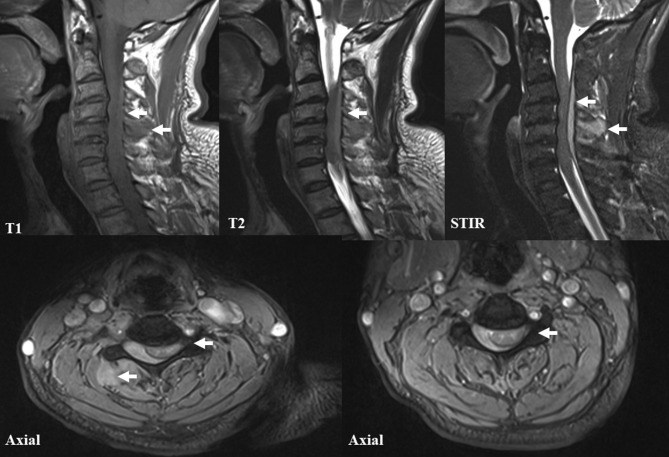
Magnetic resonance imaging (MRI) of the cervical spine. The T1-weighted, T2-weighted, short tau inversion recovery (STIR) and axial cervical MRI images demonstrated a longitudinal dorsal extradural hematoma, extending from the C2 to C7 levels. In addition, severe cord compression and related subcutaneous soft tissue hemorrhage are also noted.
